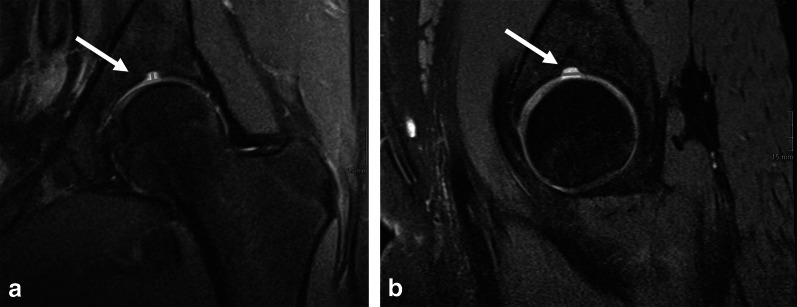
An example of the placed calipers to perform the measurements on MRI without contrast of the left hip of a 16-years-old, female participant. Proton density weighted (PD) coronal (a) with calipers to measure height and width (a), PD sagittal (b) with calipers to measure depth (b). Dimensions were 6 mm (height), 3 mm (width) mm and 7 mm (depth) (white arrows)
